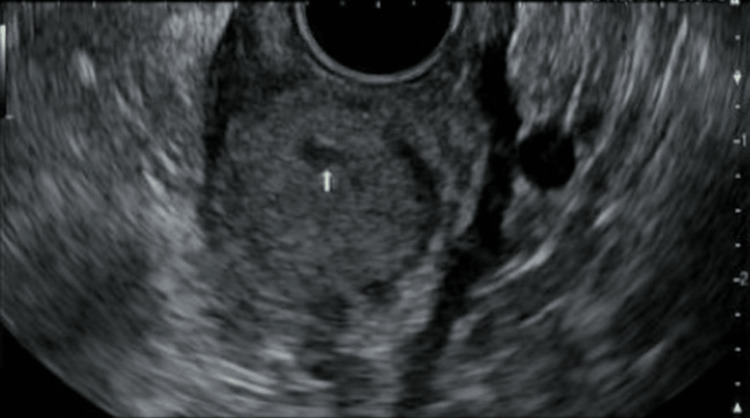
Endosonography (EUS) showing an 18.5 x 17.5 mm lesion in the gastric wall.
